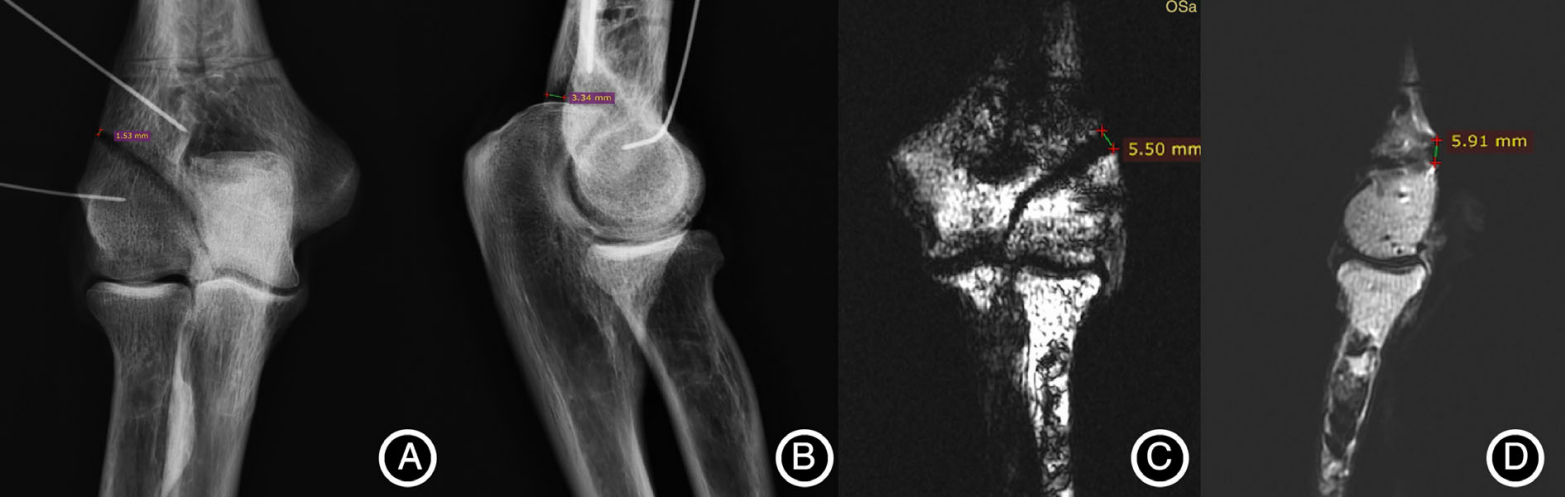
The measurement of the LFS/PFS distance of the fracture model on the X‐ray and MRI of the elbow joint. (A) Measurement of LFS distance on anterior–posterior radiograph. (B) Measurement of PFS distance on lateral elbow radiographs. (C) Measurement of LFS distance on coronal T1WI of the elbow MRI. (D) Measurement of PFS distance on sagittal MRI T2WI of the elbow joint.
